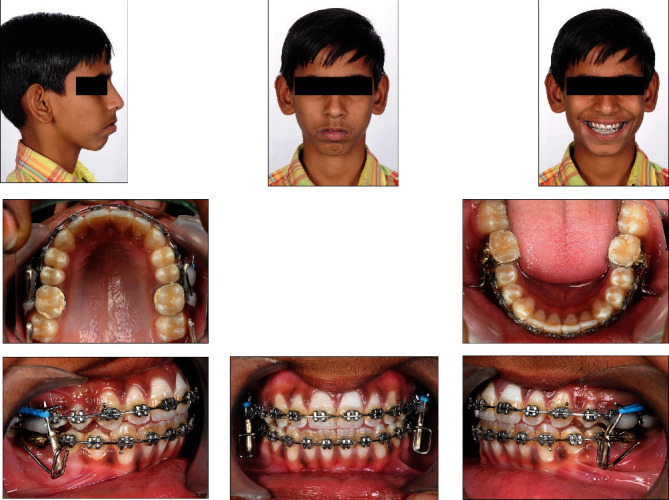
With in-house fabricated*, customized FM2 JET appliance* photographs and radiographs.
